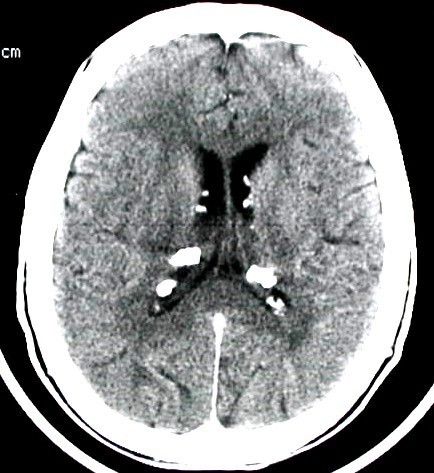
subependymal calcification as seen at the CT scan.
